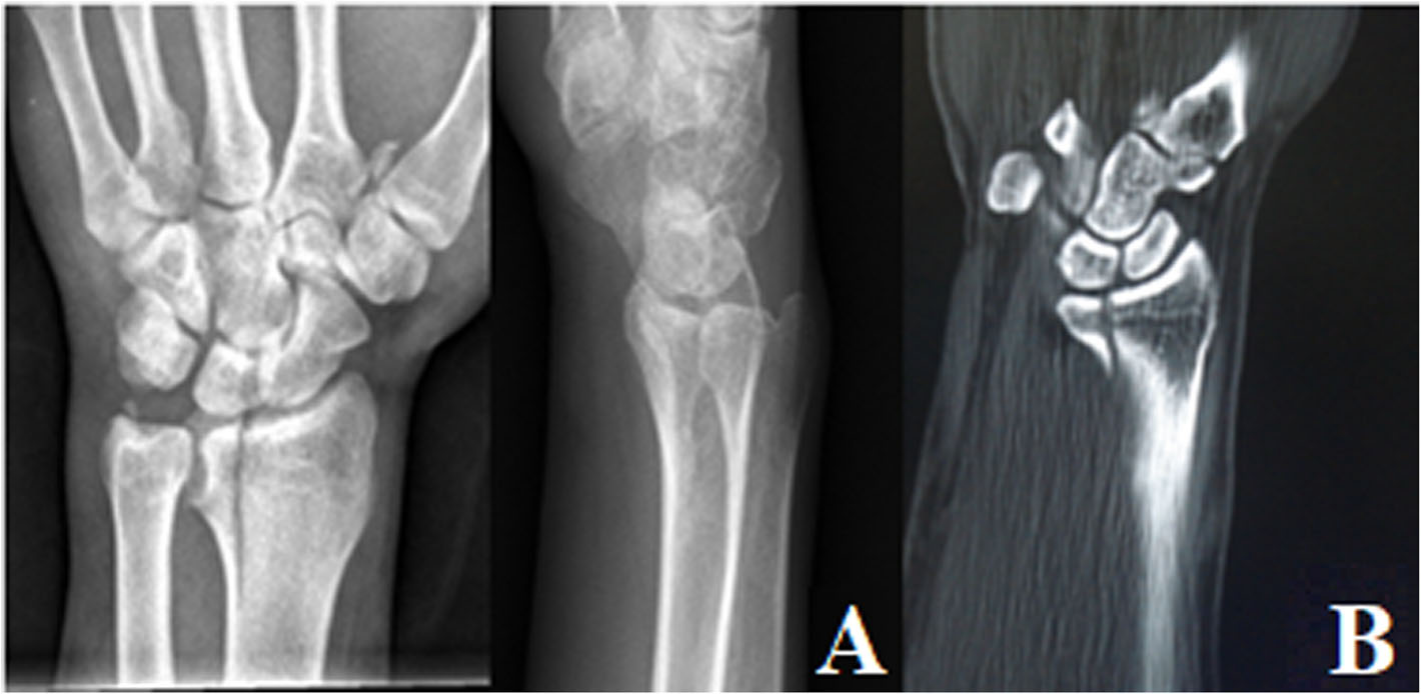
PA X-ray (a) and CT scan (b) of the spilt single-column DRDPF
